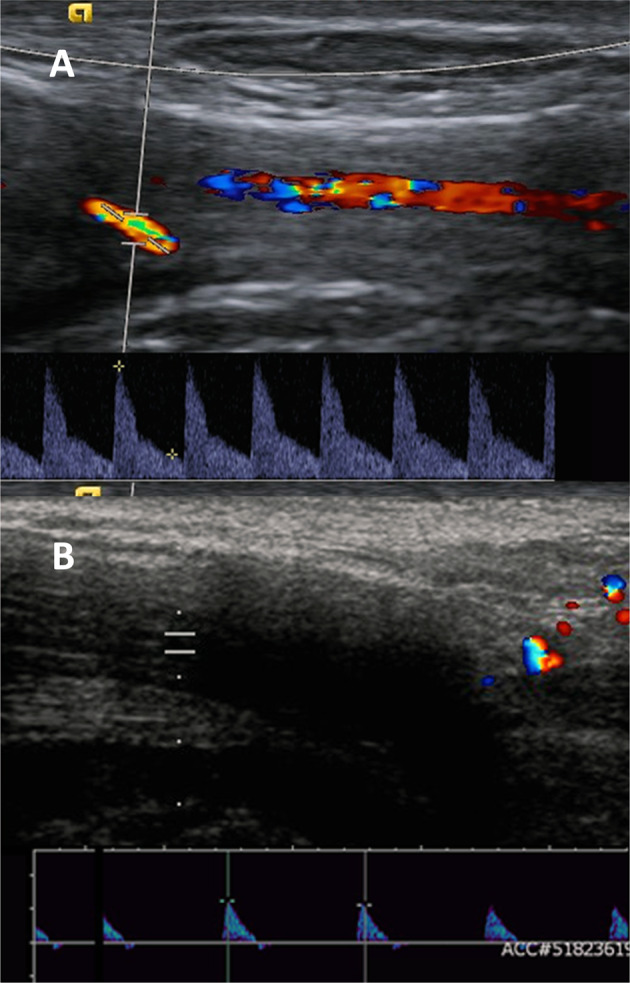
Unstimulated Penile Doppler Ultrasound findings in Groups 1 (Stuttering Priapism) and 2 (Sleep-related painful erections). A Group 1: Resting waveform in patients with stuttering priapism and multiple previous episodes of ischemia. This shows a high-flow low-resistance waveform. The peak systolic velocity is around 40 cm/s with the artery seen easily on color imaging and the forward flow in diastole make it a low resistance waveform. This is an abnormal resting waveform and is normally seen in phase two of the normal erectile cycle. B Group 2: Resting waveform in patients with SRPE and have not had an episode of ischemic priapism. This shows a low flow high resistance waveform. The peak systolic velocity is less than 10 cm/s, hence it is difficult to see the cavernosal artery on the color trace, and the lack of flow in diastole makes it a high resistance waveform. This is the resting waveform seen in normal men with a normal penis.
